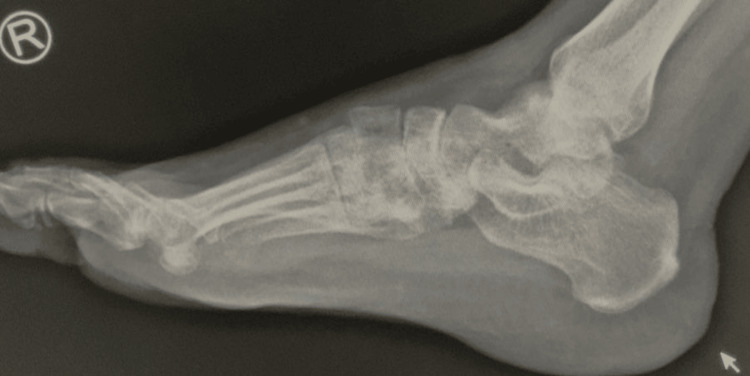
Six-month follow-up X-ray of the right foot.
